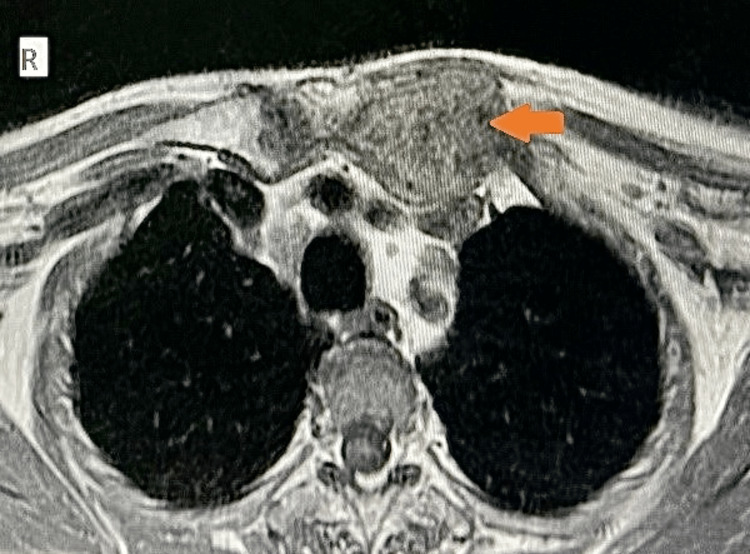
Plain MRI of the thorax axial T1-weighted image showing a relatively well-defined, altered signal intensity, heterogeneous, predominantly isointense lesion at the left sternoclavicular joint, juxta-cortical in a location involving the manubrium sterni and the medial end of the clavicle with adjacent soft-tissue components (arrow).
